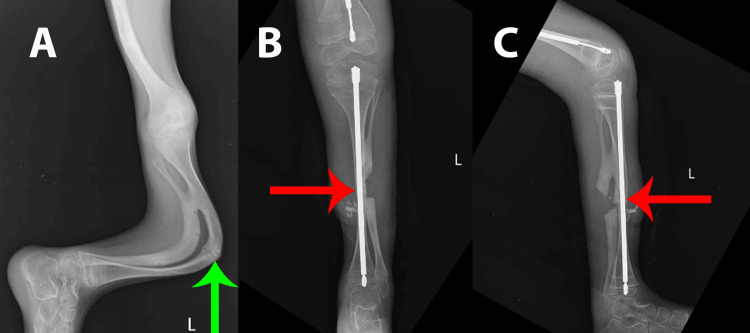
(A) Preoperative lateral X-ray of the left tibia with a significant bowing deformity, particularly at the distal third, with a sharp curvature (indicated by the green arrow). (B) Immediate postoperative X-ray with the placement of an intramedullary rod within the left tibia (indicated by red arrow). The alignment of the bone has been corrected, with the intramedullary rod stabilizing the tibia to prevent further deformity. The osteotomy site is visible, and the bone segments are aligned. (C) Another immediate postoperative X-ray from a lateral view with the intramedullary rod in place along the tibia. This view provides additional confirmation of the stability achieved postoperatively.
